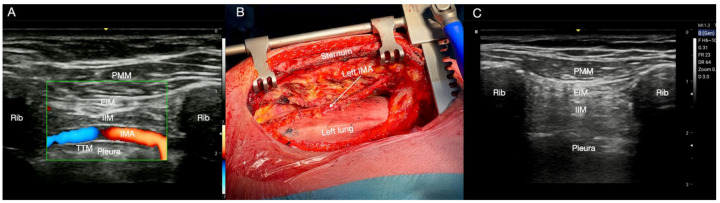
Internal mammary artery anatomy. (A) The internal mammary artery (IMA) runs between the internal intercostal and transversus thoracis muscles (IIM and TTM, respectively), as shown by the Doppler imaging; (B) IMA harvesting, (C) ultrasound image of the landmarks after IMA harvesting: note the TTM disruption rendering the transversus thoracis plane block difficult to perform. Pectoralis major muscle (PMM); external intercostal muscle (EIM).
